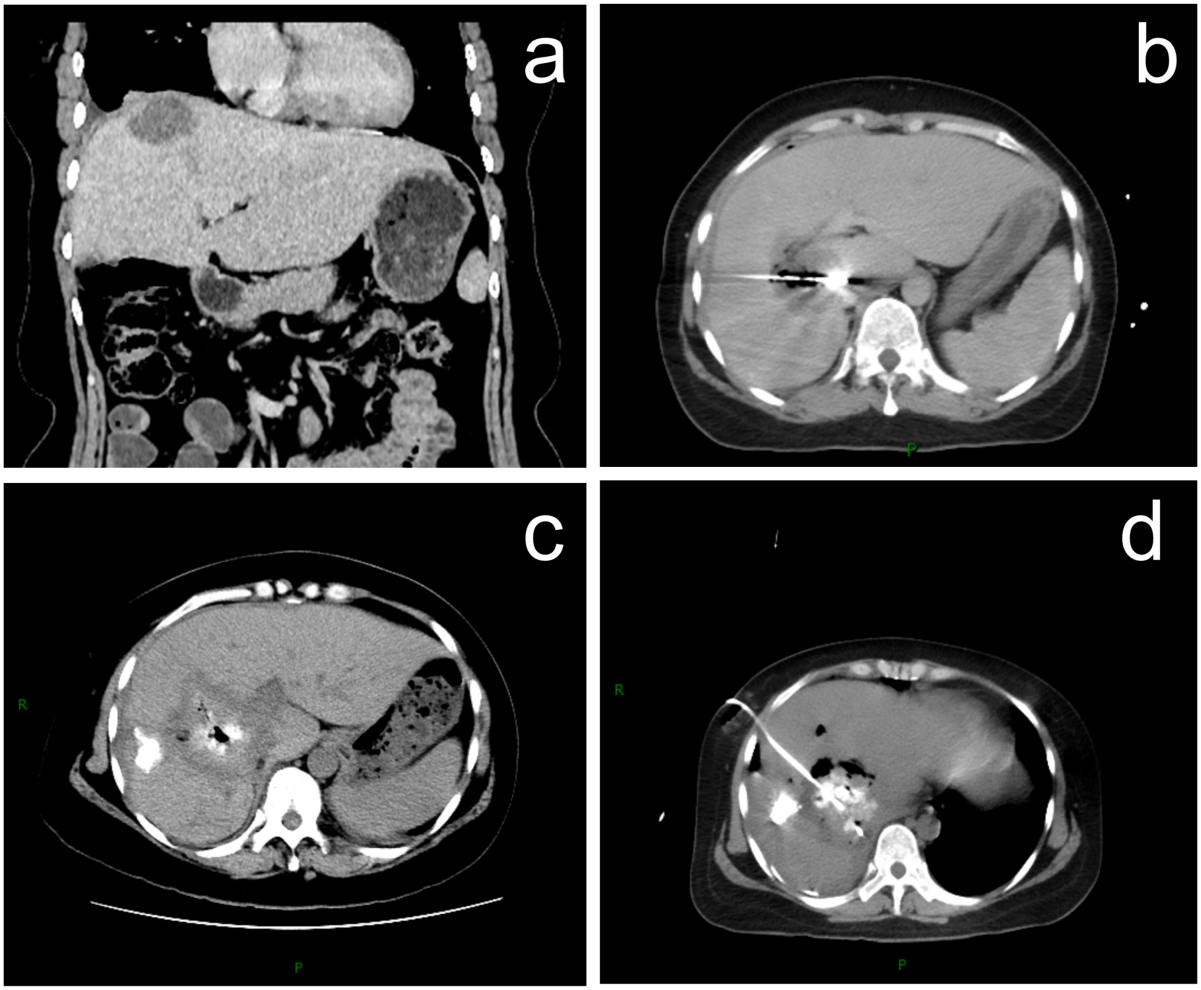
Percutaneous microwave ablation (PMWA) of liver metastases and computed tomography (CT) images of pyogenic liver abscesses (PLA). (a) Coronal CT image showing the tumor adjacent to diaphragm (red arrow); (b) PMWA procedure for liver metastasis; (c) CT image of PLA; (d) percutaneous drainage procedure of PLA.
